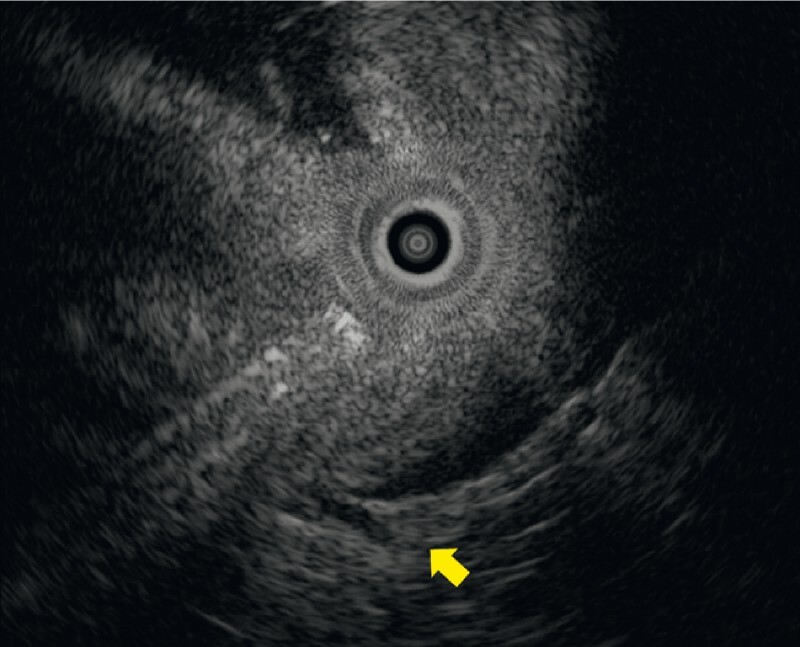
Endoscopic ultrasound image showing a partial defect of the fourth layer, which overlaps discontinuously from each side.
